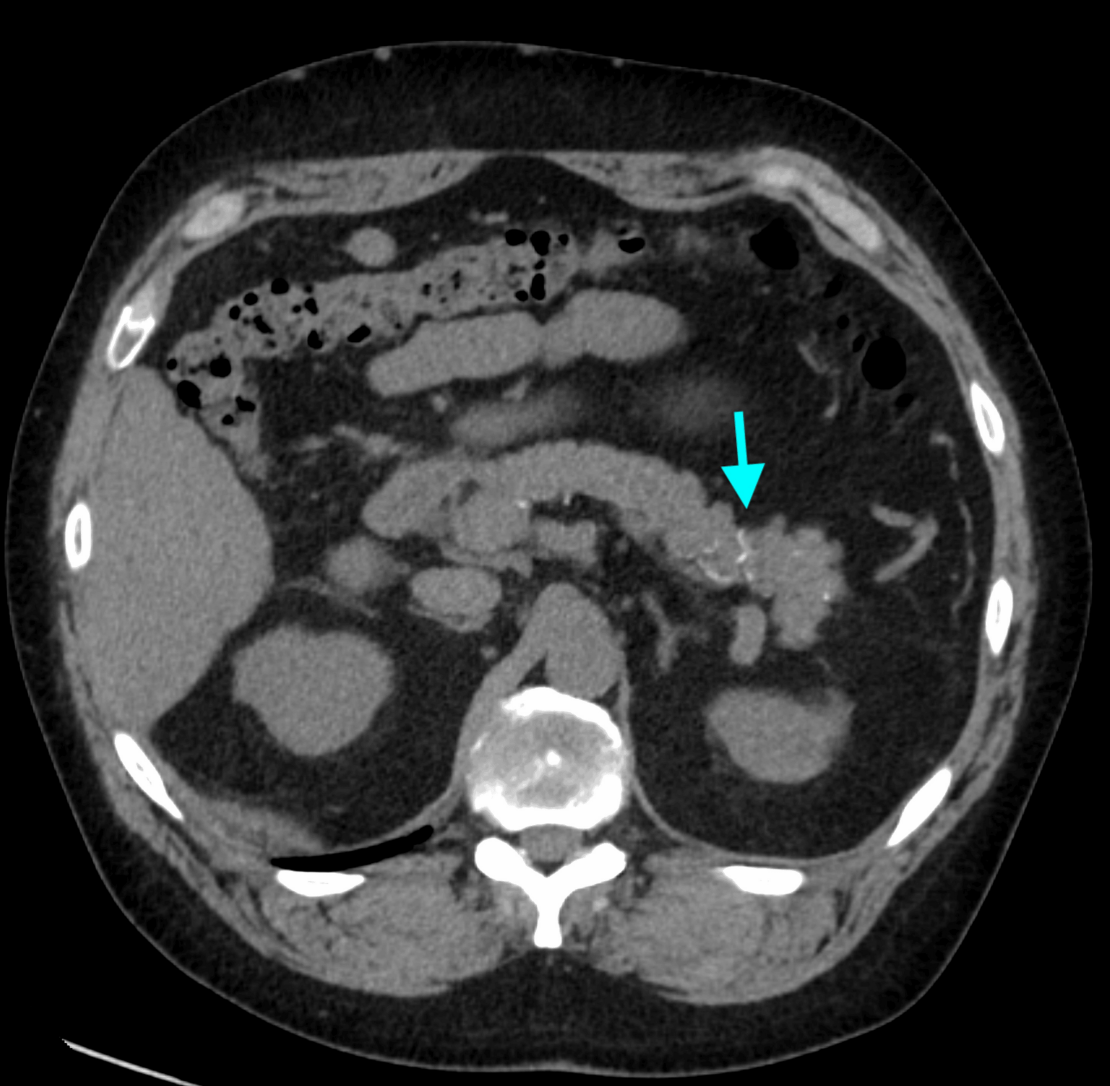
Repeat CT in 2021, four years after initial presentation, shows development of unusual small vessel calcification within the pancreatic tail (arrow).
